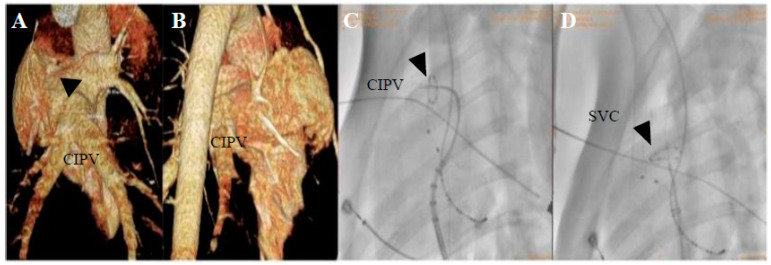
Pulmonary vein computed tomography angiography (CTA) and DSA images with PFA are shown. (A,B) CTA imaging for the CIPV anatomical measurements. (C,D) DSA is used for catheter positioning, CIPV and SVC ablations were performed during PFA.
